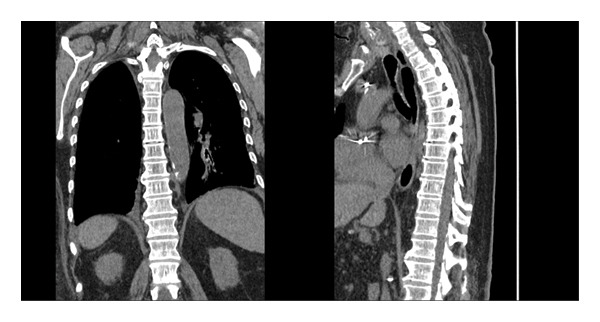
Computed tomography (CT) of the thoracic spine without intravenous contrast and sagittal and coronal reconstructions, demonstrating no evidence of osseous involvement.
